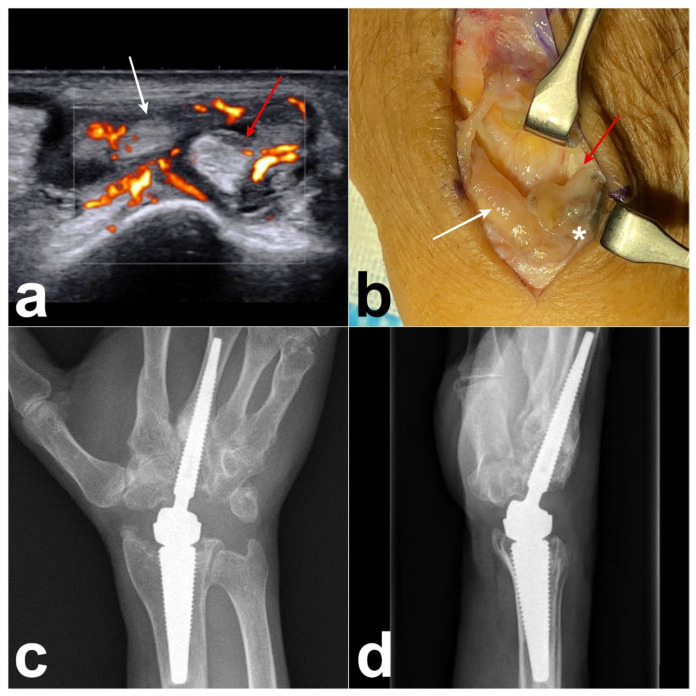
Case 2. (a) Ultrasonography reveals tenosynovitis around the EPL (white arrow), ECRB (red arrow), and ECRL. (b) Preoperative photograph showing the presence of tenosynovitis over the EPL (white arrow) and ECRB (red arrow), and associated with metallosis (white asterisk). (c,d) Postoperative radiograph showing the Motec wrist prosthesis with PEEK cup.
